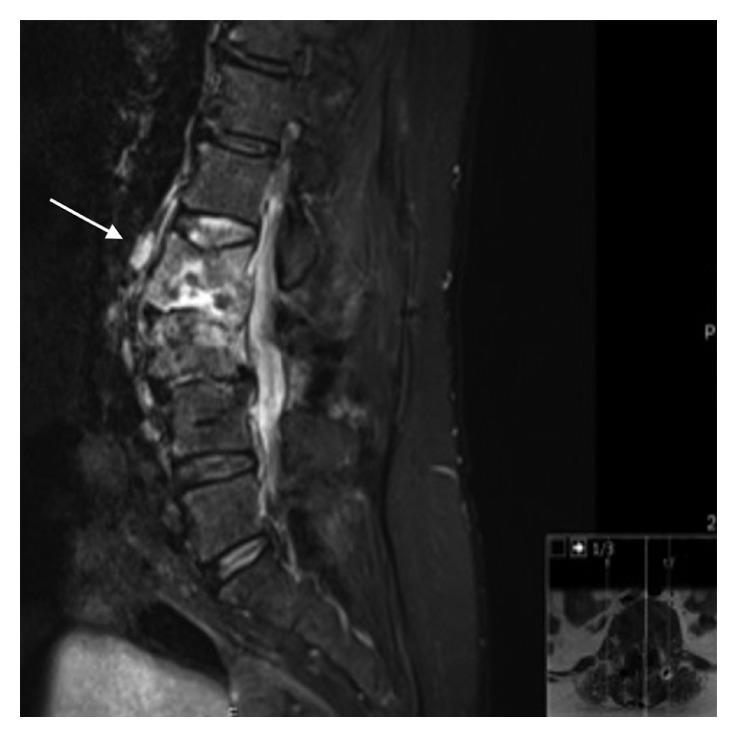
Case 2: sagittal T2-weighted image showing lytic lesions at L2 and L3 vertebral bodies, intervertebral disc destruction, anterior epidural abscess (white arrow), and scoliotic deformity centered in L2-L3.
